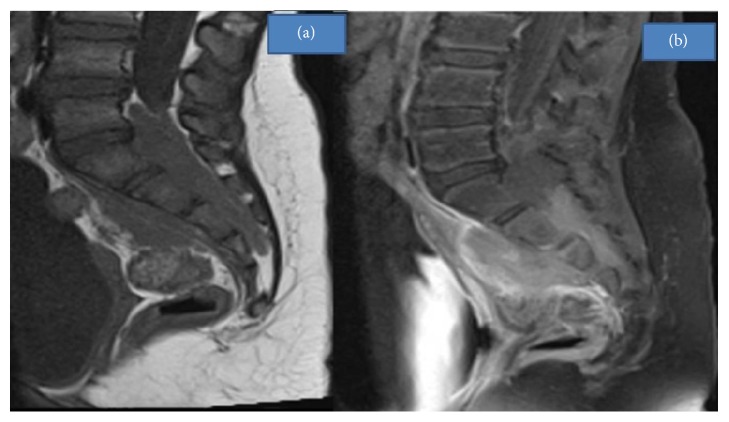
(a) Sagittal T1-weighted MRI shows a hypointense intracanalar and presacral infiltrating mass. (b) Postcontrast T1-weighted sagittal image with fat suppression shows heterogeneous enhancement of the mass and sacral vertebrae.
